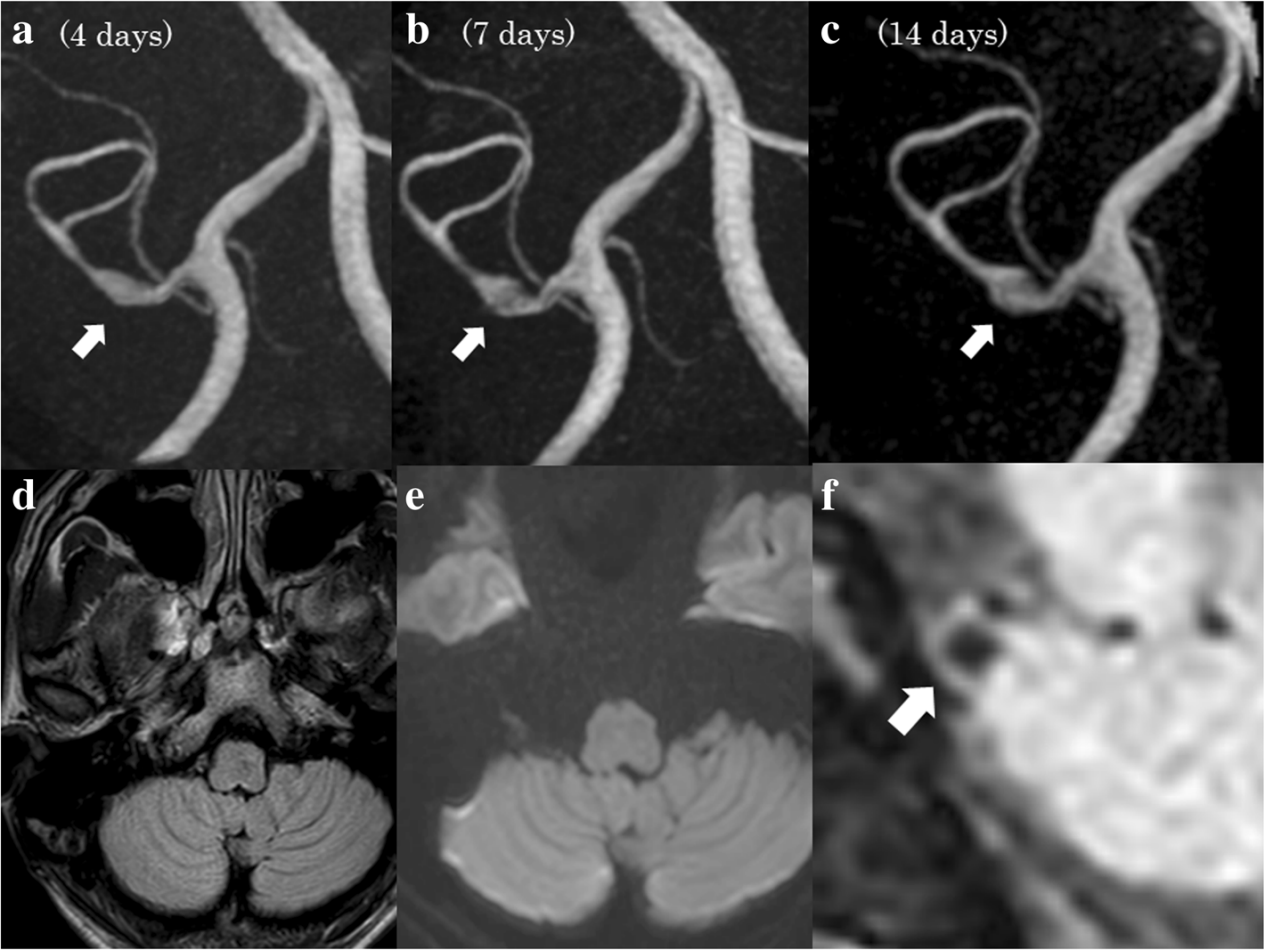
a, b and c are MRA (MIPs). A (4 days after onset) shows ‘pearl and string sign’ like finding of the patient’s right PICA. The PICA inner diameters are growing gradually (7 days later) (b) and (14 days later) (c). The FLAIR and DWI shows no hemorrhages and infarctions, respectively (d and e). T1-weighted vessel wall image depicts no apparent intramural hematomas of the patient’s right PICA (F). (All arrows indicate the affected PICAs)
